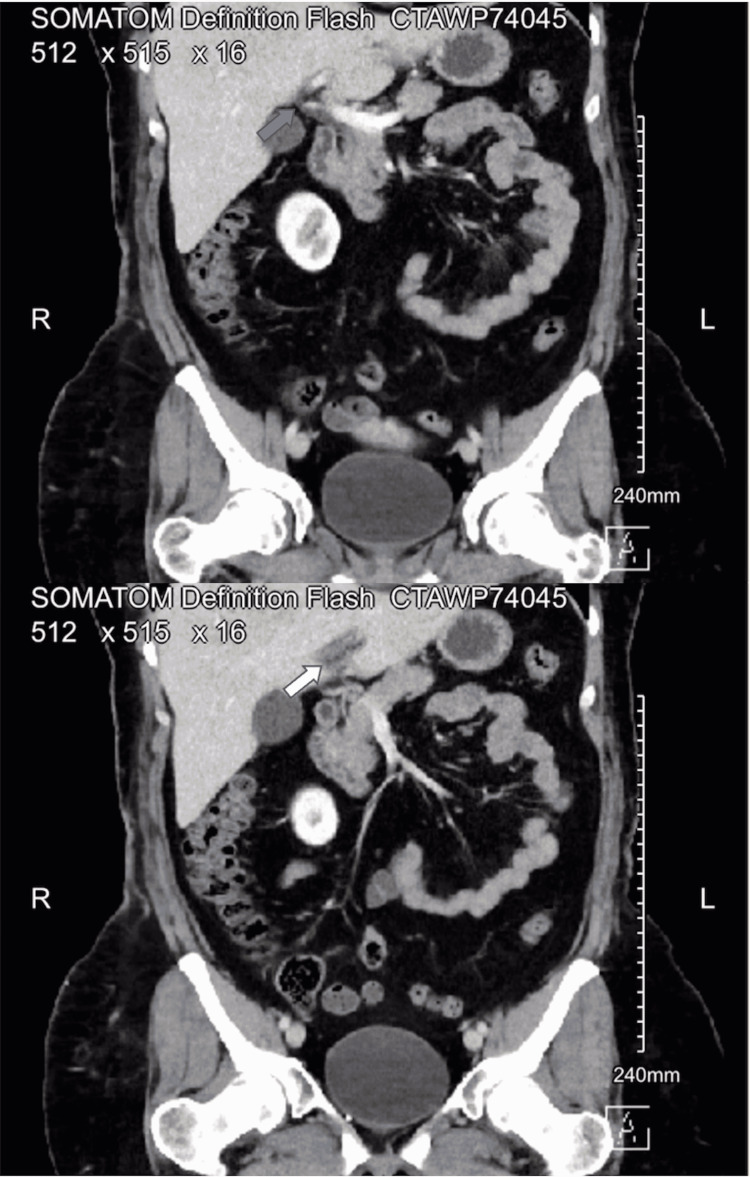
CT abdomen/pelvis (coronal view) Coronal view of proximal (grey arrow) and distal portal vein thrombosis (white arrow)
